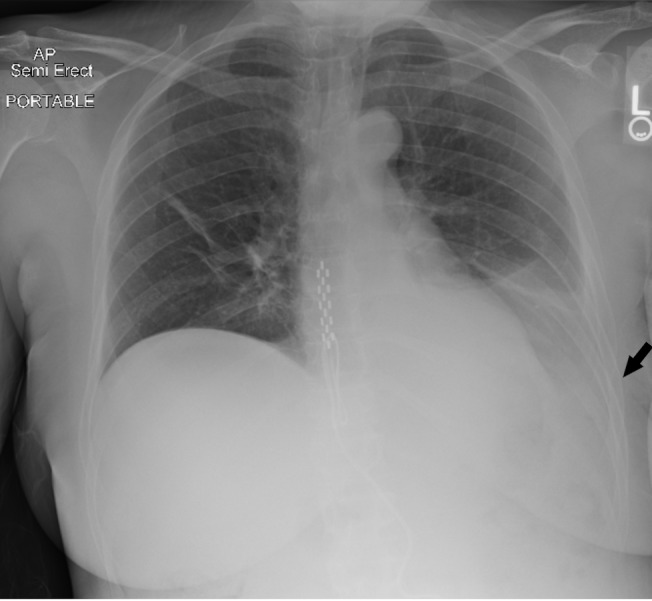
Chest X-ray on postoperative day 3 showing left-sided pleural effusion.
